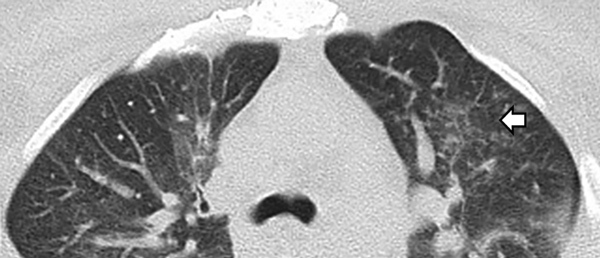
Computed tomography scan of the chest in a patient with *Mycobacterium obuense* pneumonia, Mexico, showing air space infiltration in the left parahilar and a tree bud pattern in the left upper lobe (arrow), as well as bilateral interstitial thickening and ground glass opacities.
